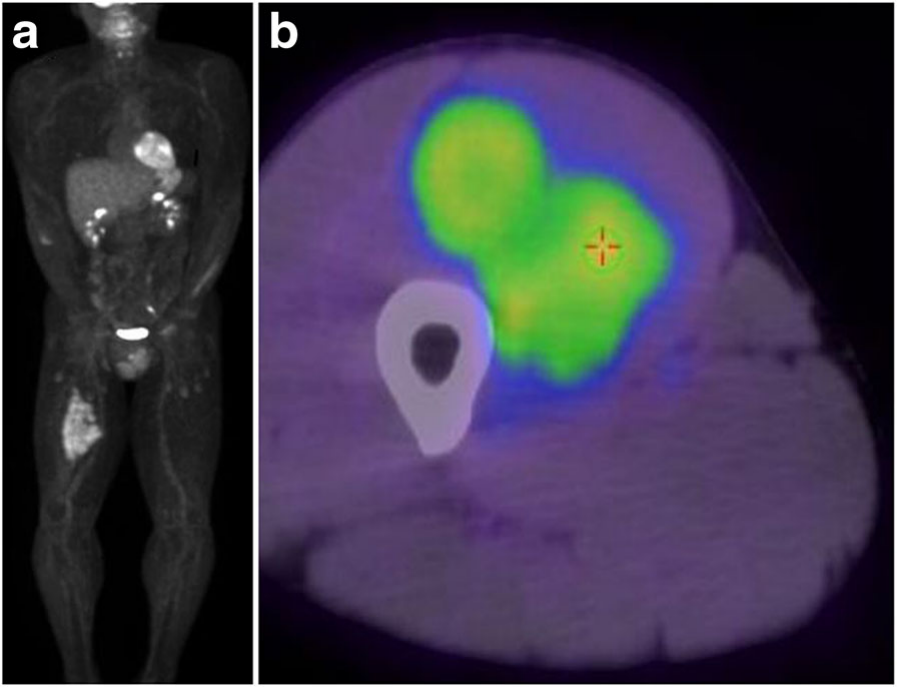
a Positron emission tomography-computed tomography reveals no obvious distant metastases. b An axial image with the maximum standardized uptake value of 3.1 (at the crosshairs)
